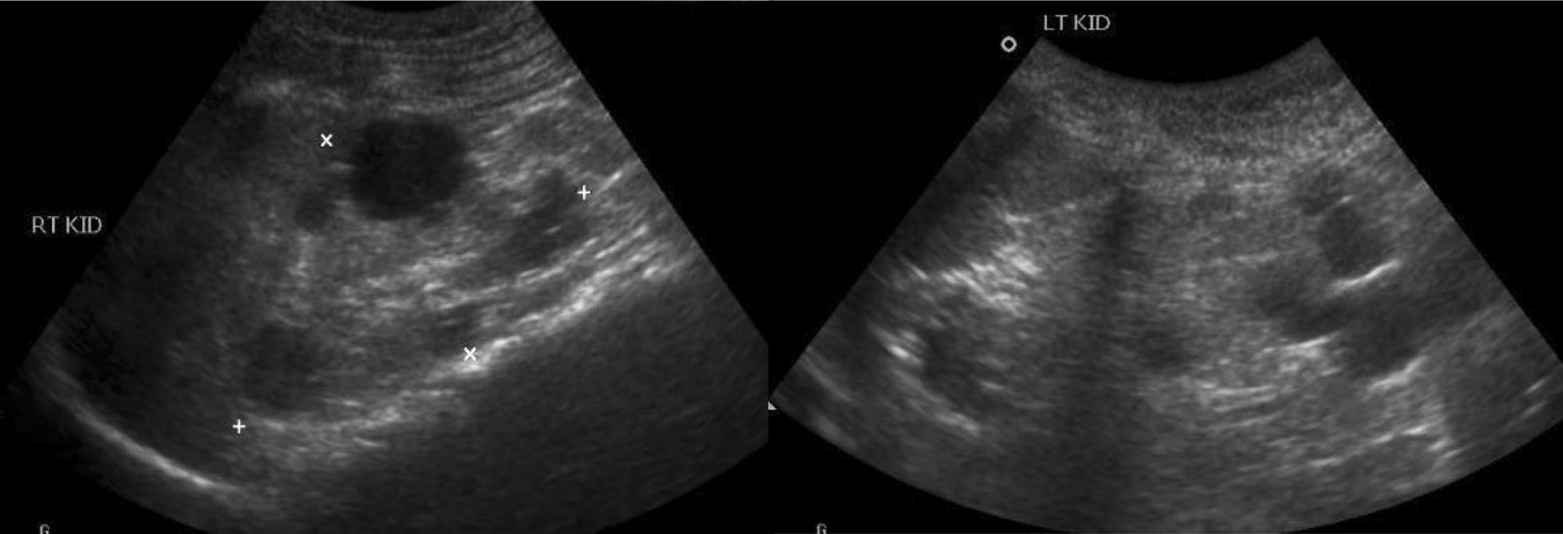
Renal ultrasound, age 7 years. Both kidneys are 9 × 3.5 cm. Multiple cortical cysts of variable size are seen in both kidneys
